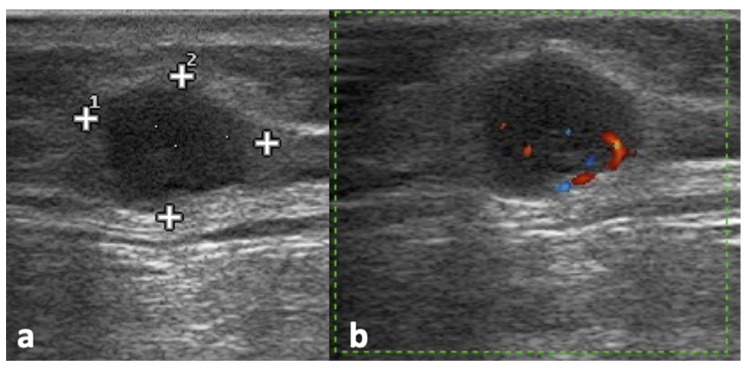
Left subscapular lymphadenopathy representing the recurrence of a cutaneous melanoma on the trunk. The B-Mode US scan (a) shows an oval, markedly hypoechoic, inhomogeneous lymph node with irregular borders. The color Doppler scan (b) shows prevalent peripheral flow signals.
